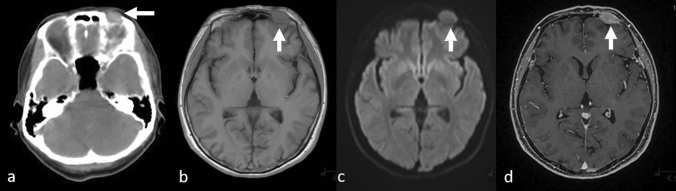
Localized osteolytic skull metastases in a patient with breast cancer. Computed tomography a shows osteolytic bone metastasis in the frontal bone (arrow); T1-weighted image b shows the tumor as a “gray” mass (arrow), while a diffusion-weighted image c shows it as a hyperintense area (arrow) and contrast-enhanced 3D fat-suppressed T1-weighted image d shows it as a homogeneously enhanced mass (arrow)
